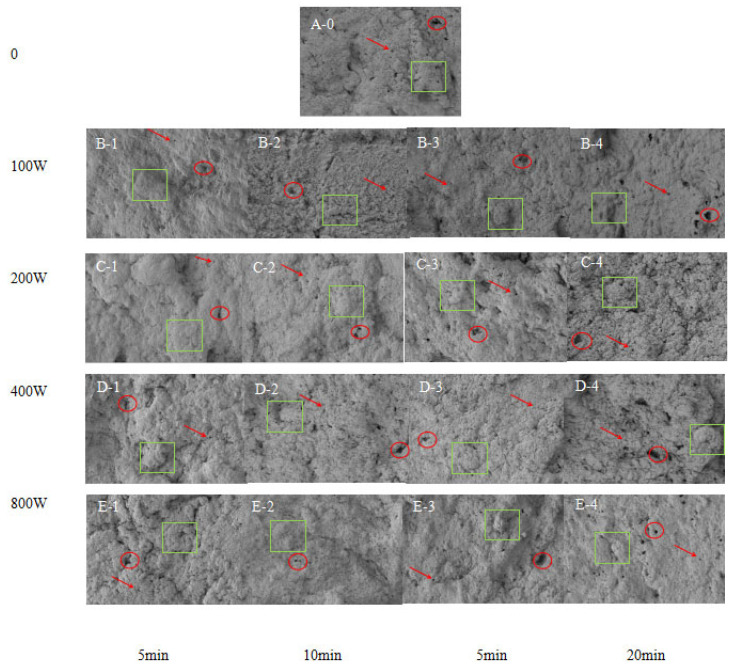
Effects of ultrasonic treatment on the microstructure of surimi gels. (A-0): untreated control. (B-1,B-2,B-3,B-4): 100 W (5 min, 10 min, 15 min, 20 min); (C-1,C-2,C-3,C-4): 200 W (5 min, 10 min, 15 min, 20 min); (D-1,D-2,D-3,D-4): 400 W (5 min, 10 min, 15 min, 20 min); (E-1,E-2,E-3,E-4): 800 W (5 min, 10 min, 15 min, 20 min). 0 min/w: untreated control. Large protein aggregates: green box; irregular pores: red circle; uniformly sized pores: red arrow.
